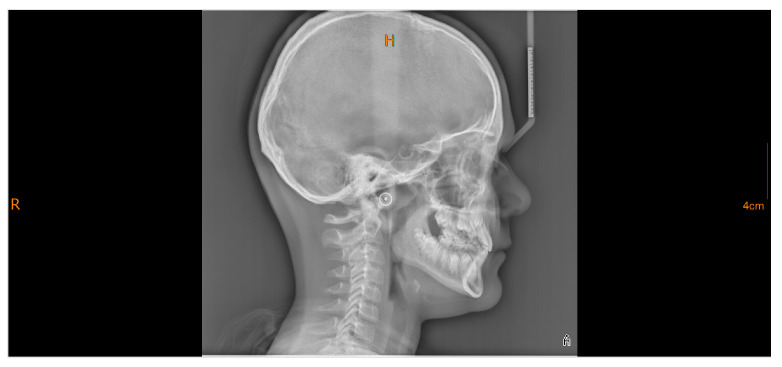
Lateral cephalometric radiograph acquired using a hybrid imaging device (combined panoramic/cephalometric/CBCT unit). Note the distinct radiopaque target marker (outer circle with central point) overlying each external acoustic meatus, with the acquisition parameters of 90 kVp, 15 mA, and 0.8 s indicating precise alignment of the ear rods and correct patient positioning without tilt or rotation.
